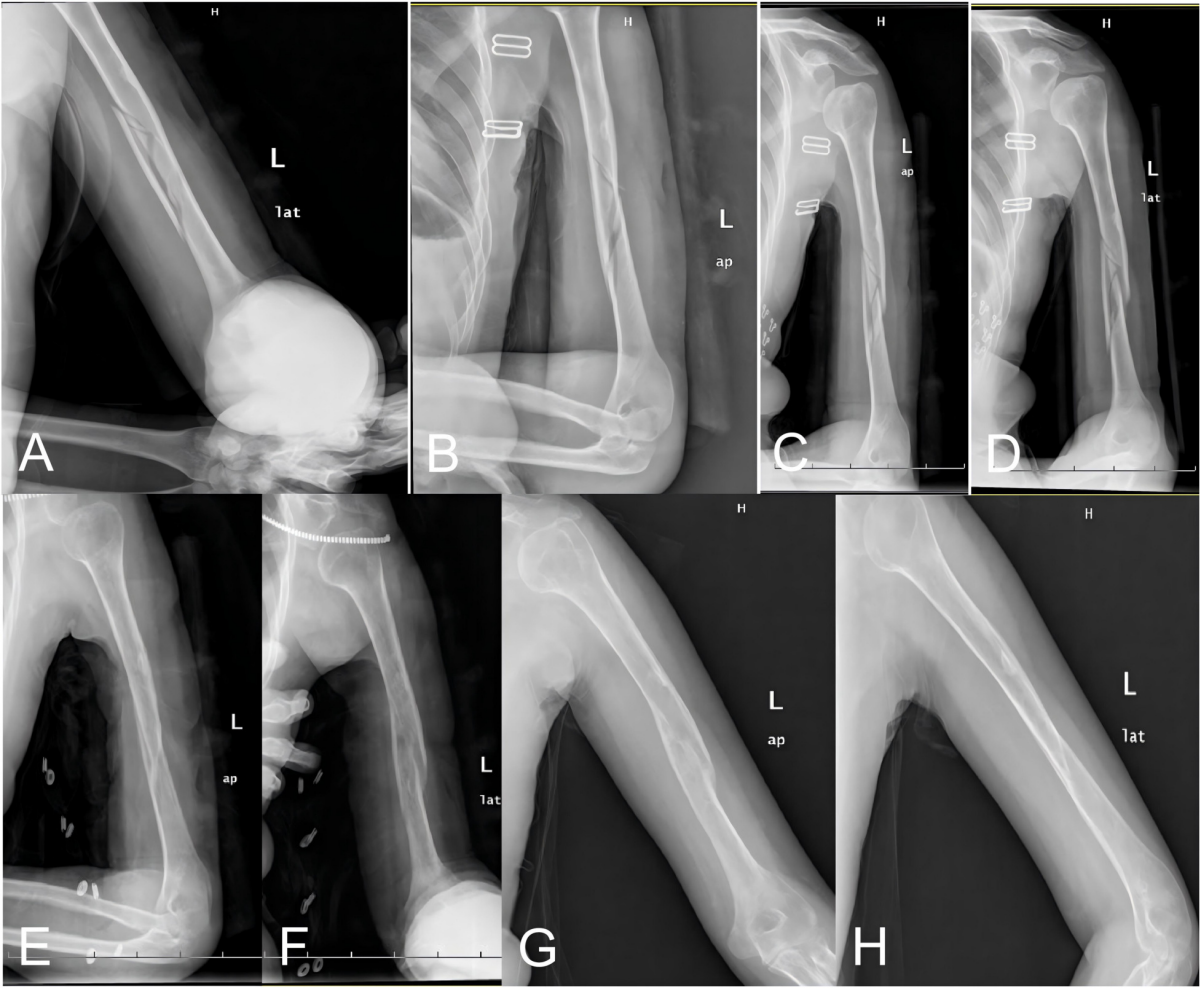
35-year-old female with a left humeral shaft fracture (AO/OTA C1). (A,B) Initial anteroposterior and lateral radiographs. (C,D) Post-reduction radiographs following splint application. (E,F) Follow-up radiographs at 10 weeks demonstrating callus formation. (G,H) Final follow-up radiographs showing cortical continuity and medullary canal patency.
